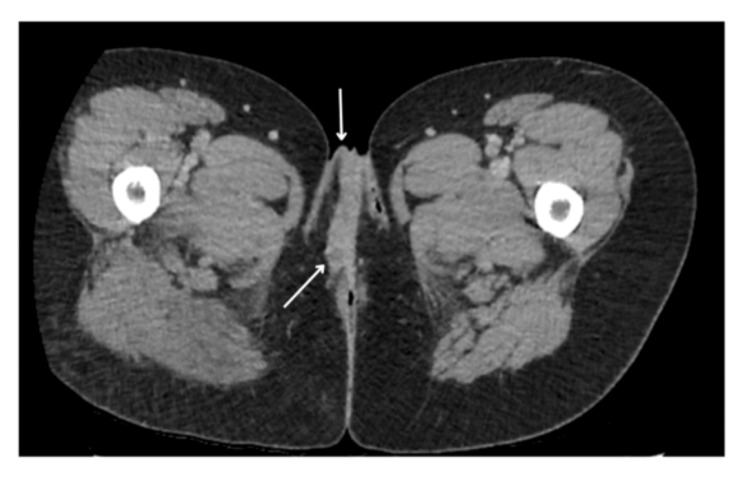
Axial post-contrast venous phase CT scan of the pelvis following presentation with complications post-embolisation. Thickening of the right labia consistent with oedema and inflammation (arrows)
